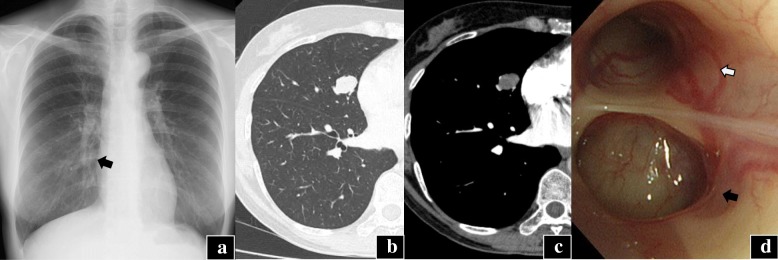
Medical imaging findings of the nodule. a Chest X-ray reveals a 2-cm shadow in the right middle lung field (black arrow). b, c CT scan reveals an 18-mm lobulated nodule. d Bronchoscopy shows the endobronchial nodule reducing the lumen of right B^5^a sub-segmental bronchus (black arrow) and the remaining patency of the B^5^b sub-segmental bronchus (white arrow)
